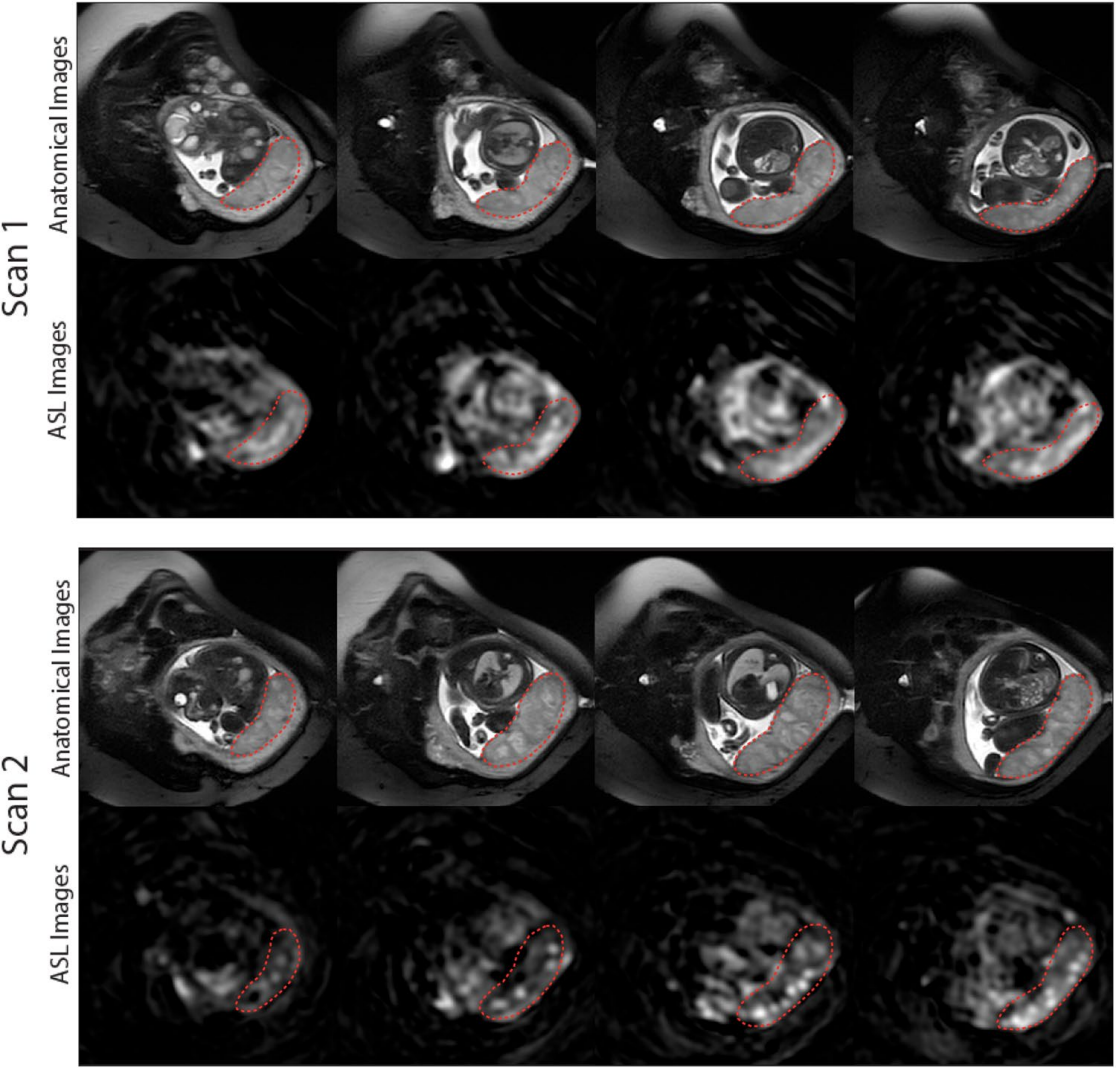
ASL and anatomical MR images of a pregnant woman with fetal CHD (diagnosis: HLHS) in the first (GA: 29 weeks) and follow-up (GA: 34 weeks) MRI scans. The placenta is delineated with the dotted line on each image. In the follow-up scan, ASL signal decreased in the regions outside lobules within the placenta, leading to increased regional variation of placental perfusion.
